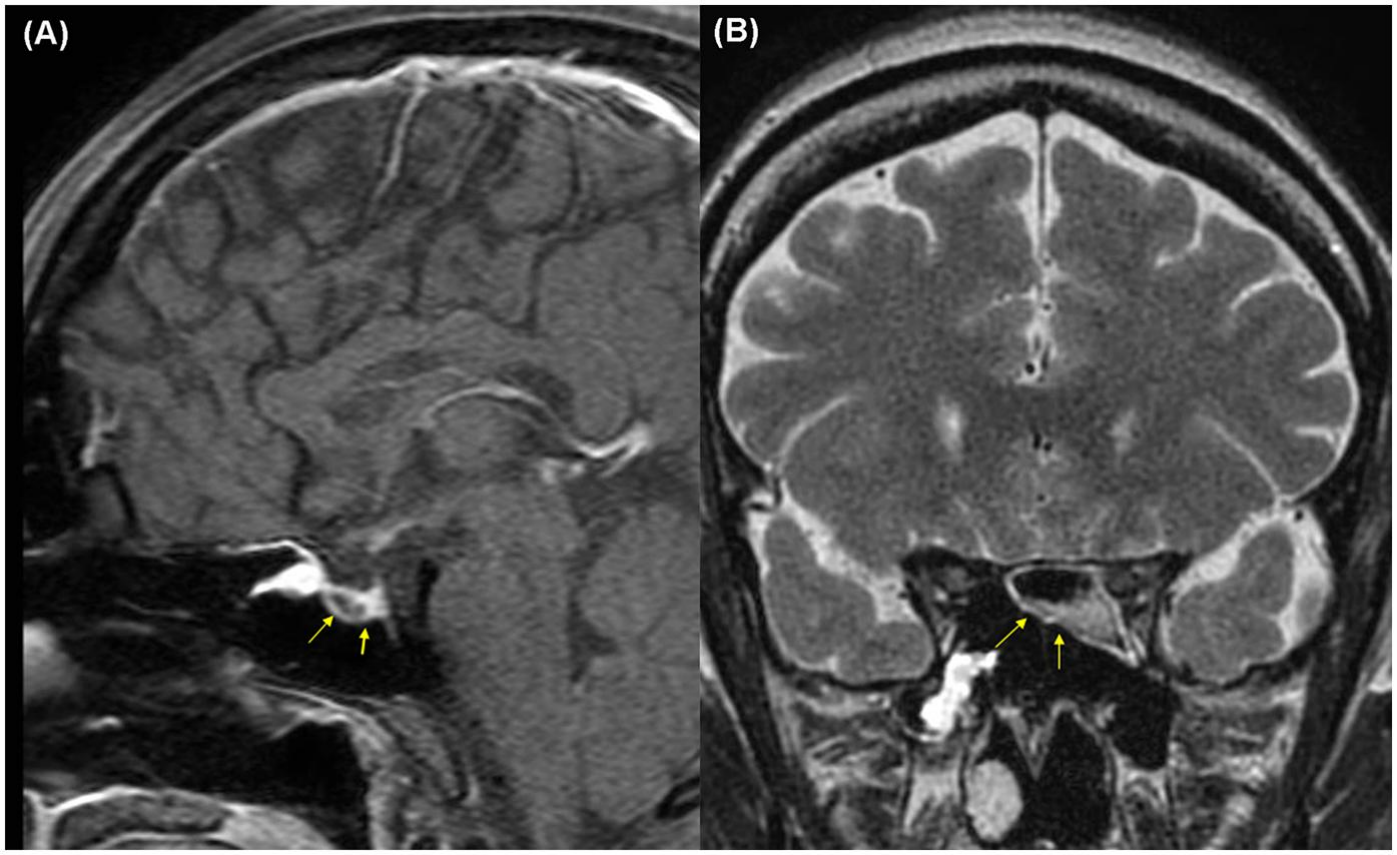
Brain magnetic resonance imaging on postpartum day 9 demonstrating hemorrhagic necrosis of the pituitary gland (yellow arrows): (A) sagittal T1-weighted image, (B) coronal T2-weighted image.
